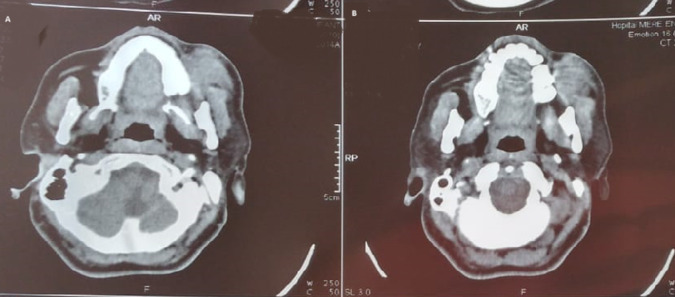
computed tomography scan revealing left maxillary mass with osteolytic features in Rosai-Dorfman disease
